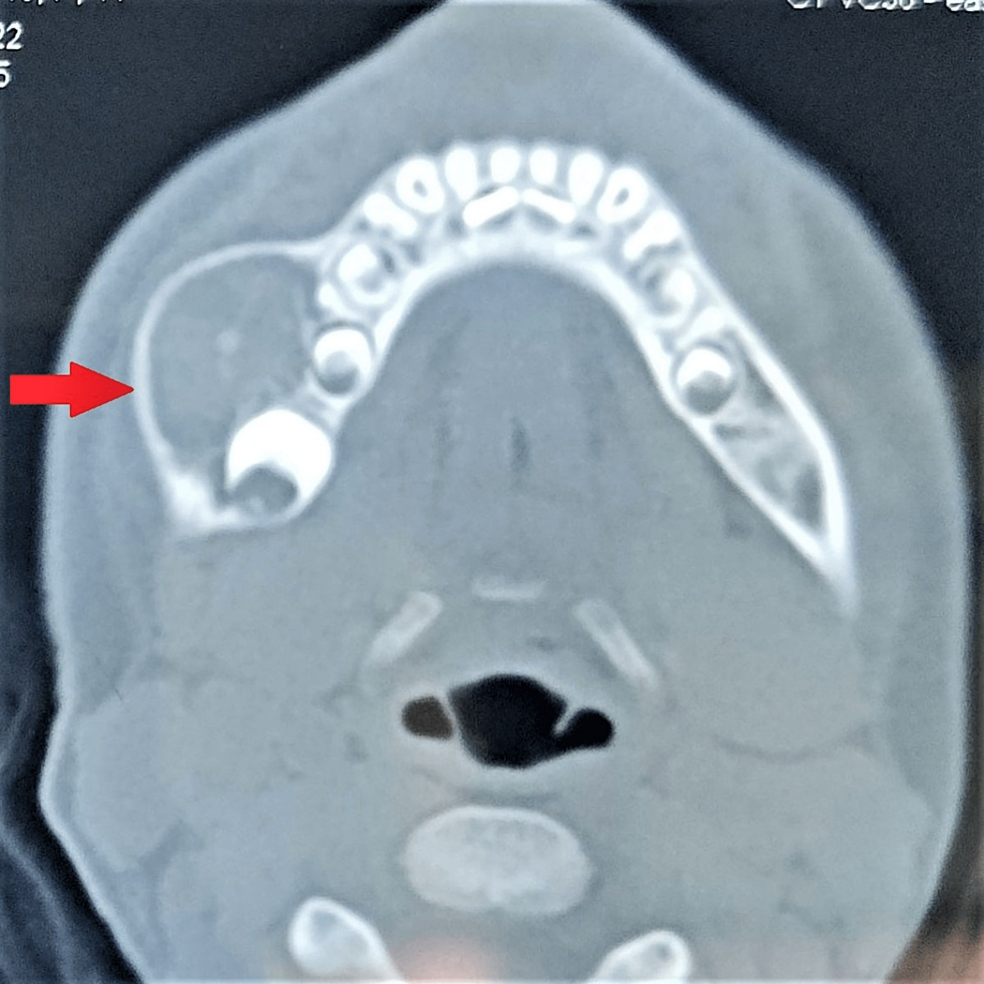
CT face plain axial section On CT face plain axial section bone window, a well-defined expansile lytic lesion was noted arising from the angle of the mandible on the right side, extending anteriorly till the deciduous molar tooth. Significant cortical thinning along with buccal expansion was noted at the perilesional area. No evidence of an obvious cortical breach was noted.
